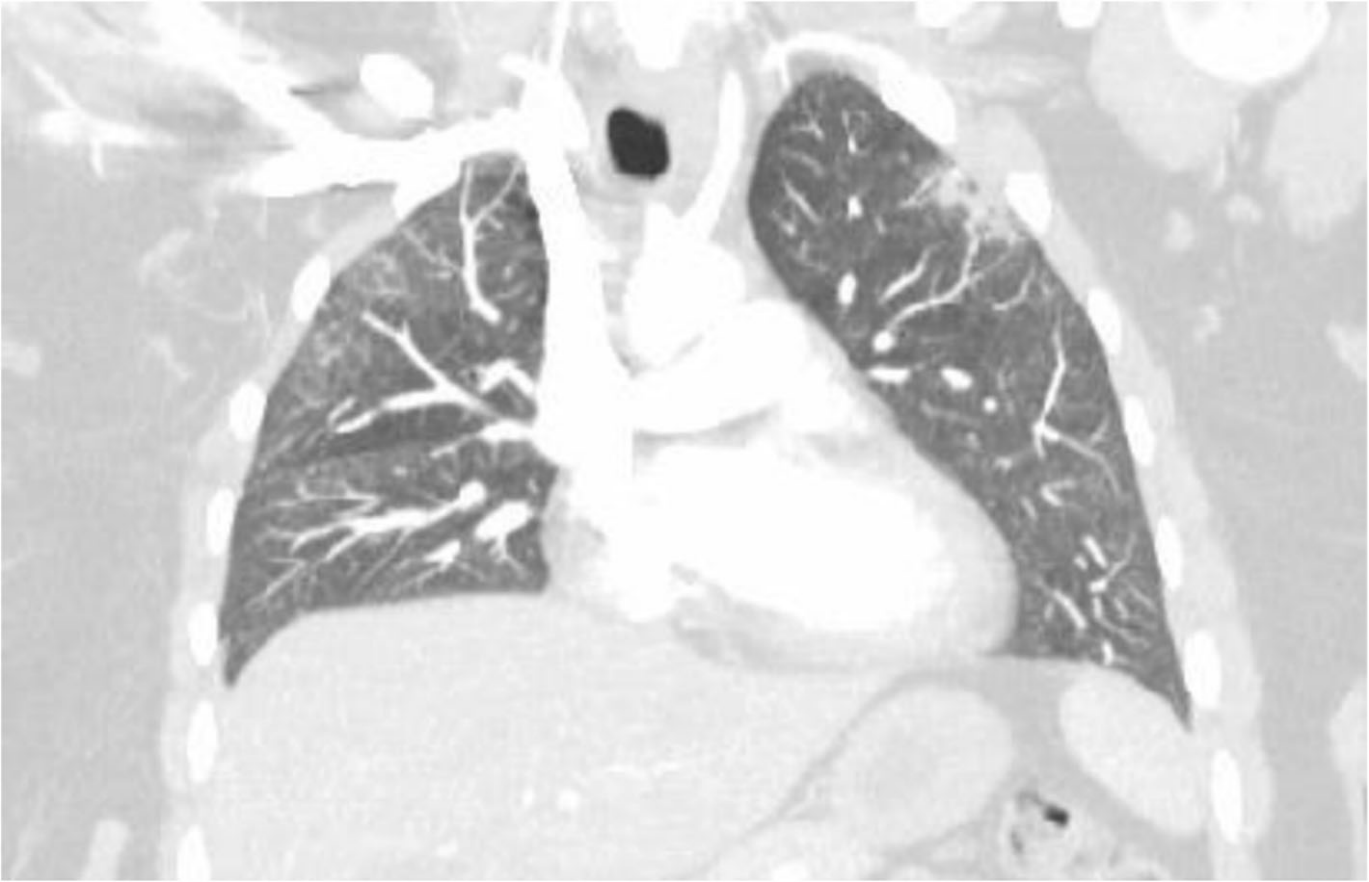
Initial CTA suggesting pneumonia and ground-glass opacities
